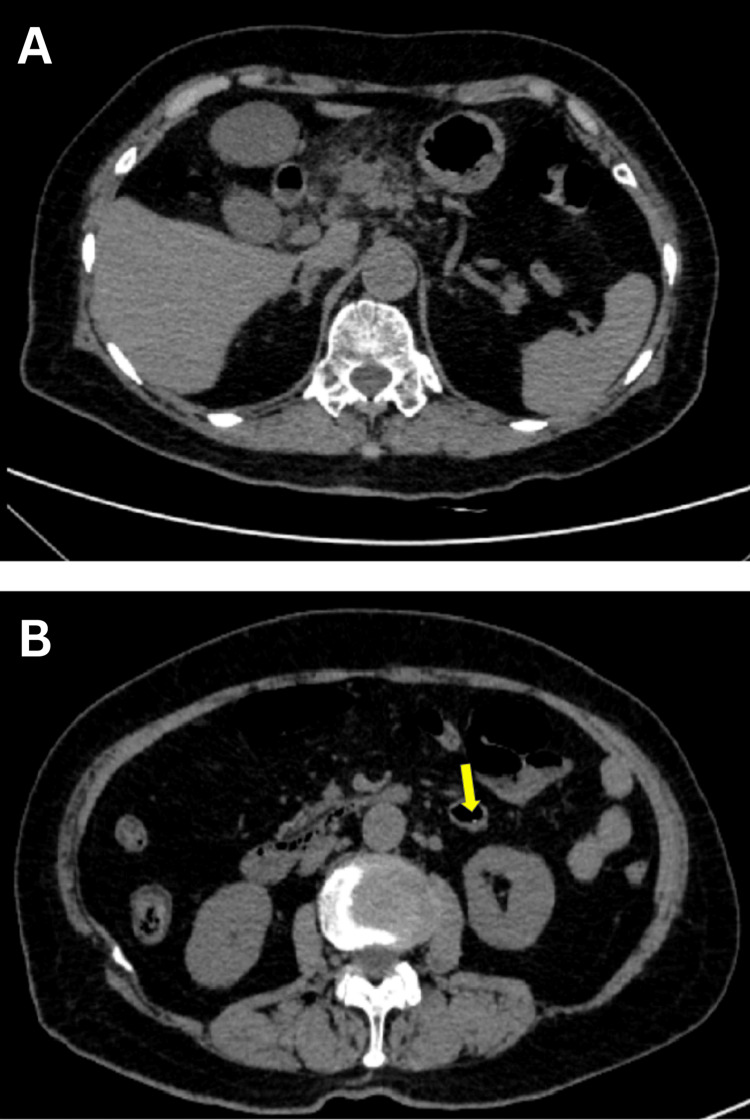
Repeated CT image 48 hours later showing the fishbone no longer in the pancreas, having migrated into the intestinal lumen (A) Upper abdominal CT image showing no fishbone in the gastric antrum and pancreas. (B) Abdominopelvic CT image showing the fishbone in the intestinal lumen. CT: computed tomography
